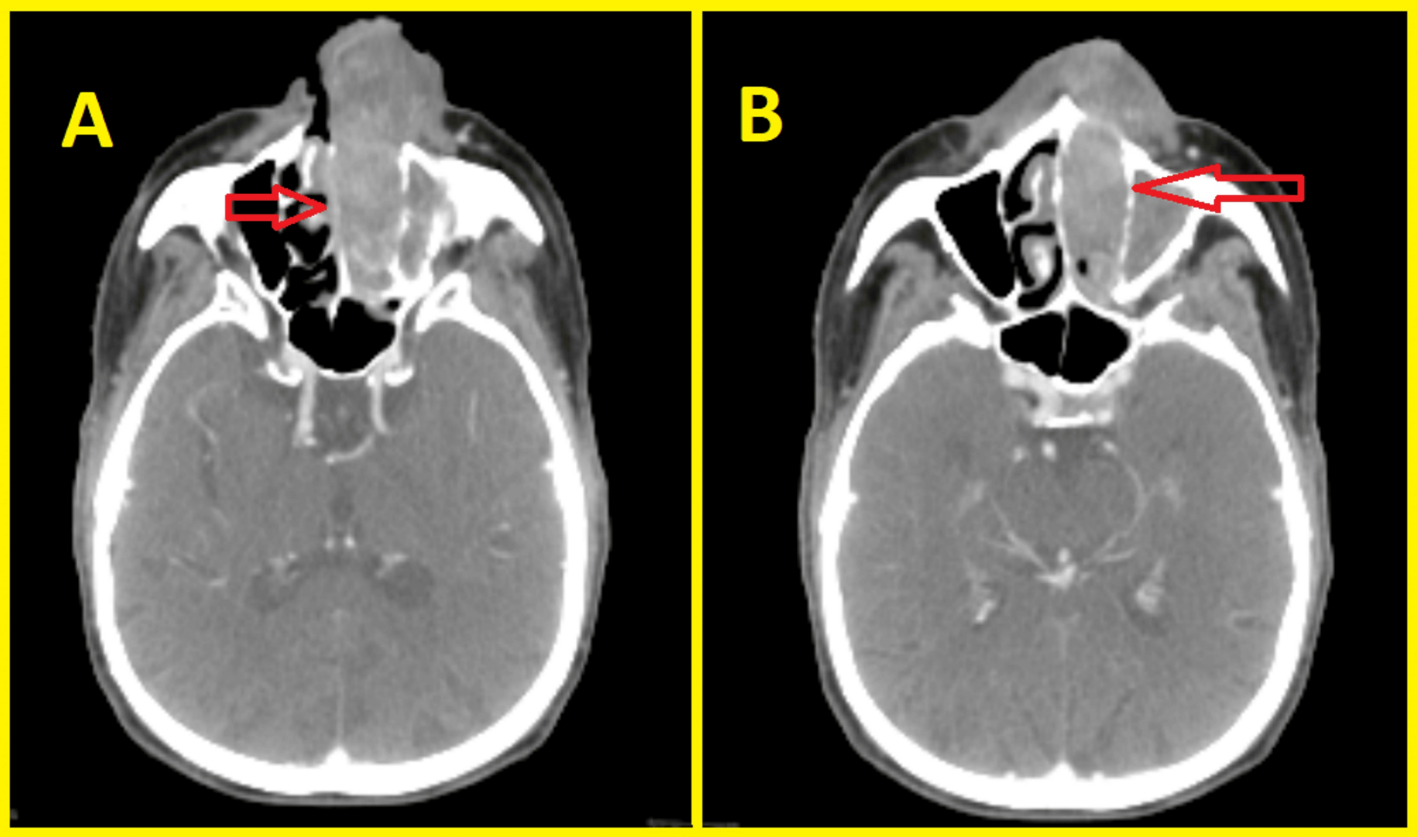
CT scan showing an expansive mass occupying the left nasal cavity and extending to the nasal septum (A), the maxillary sinus, the nostril anteriorly, and the choanae posteriorly (B) (red arrows).
